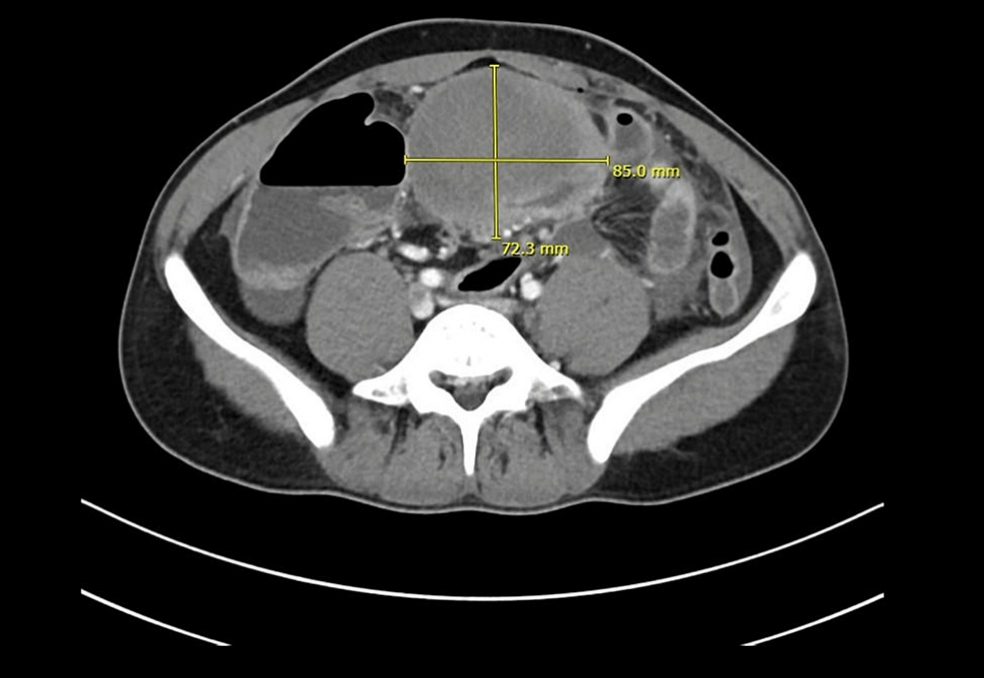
CT abdomen axial view showing the presence of a large mass in the lower abdomen CT, computed tomography
